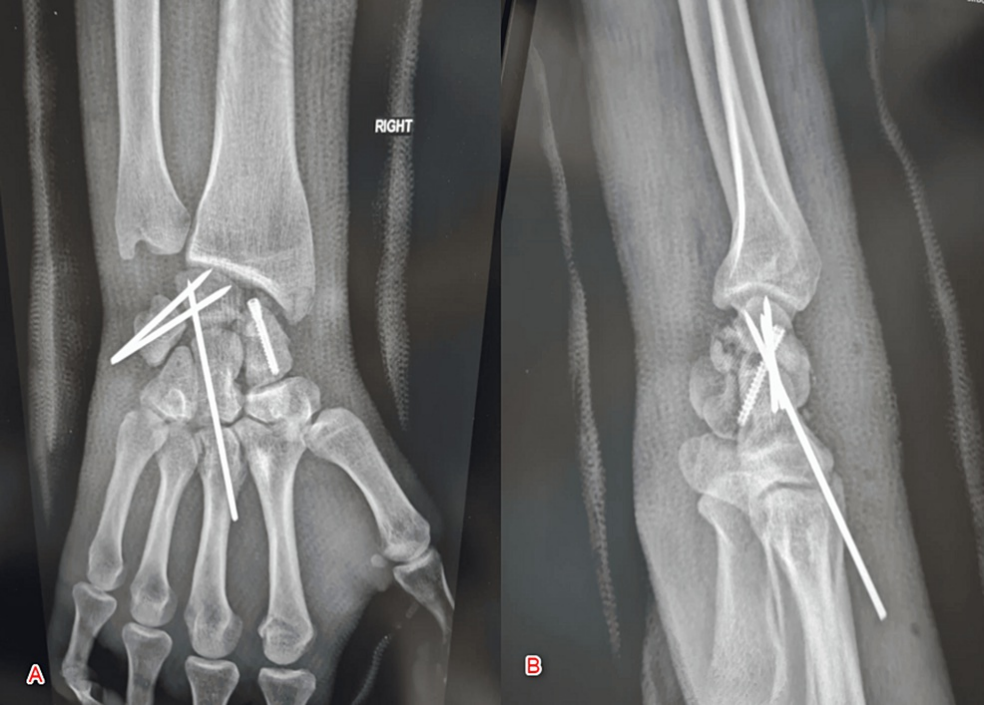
Postoperative X-ray showing stabilization of the reduction with two K-wires, reduction of the scaphoid fracture, and fixation with one screw (Herbert screw) A: Anteroposterior view; B: Lateral view
